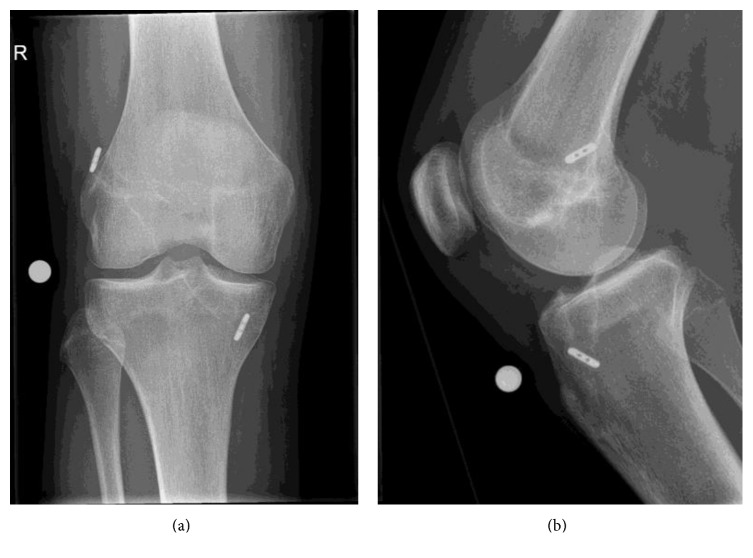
(a) and (b) Postoperative X-rays after arthroscopic ACL reconstruction.
